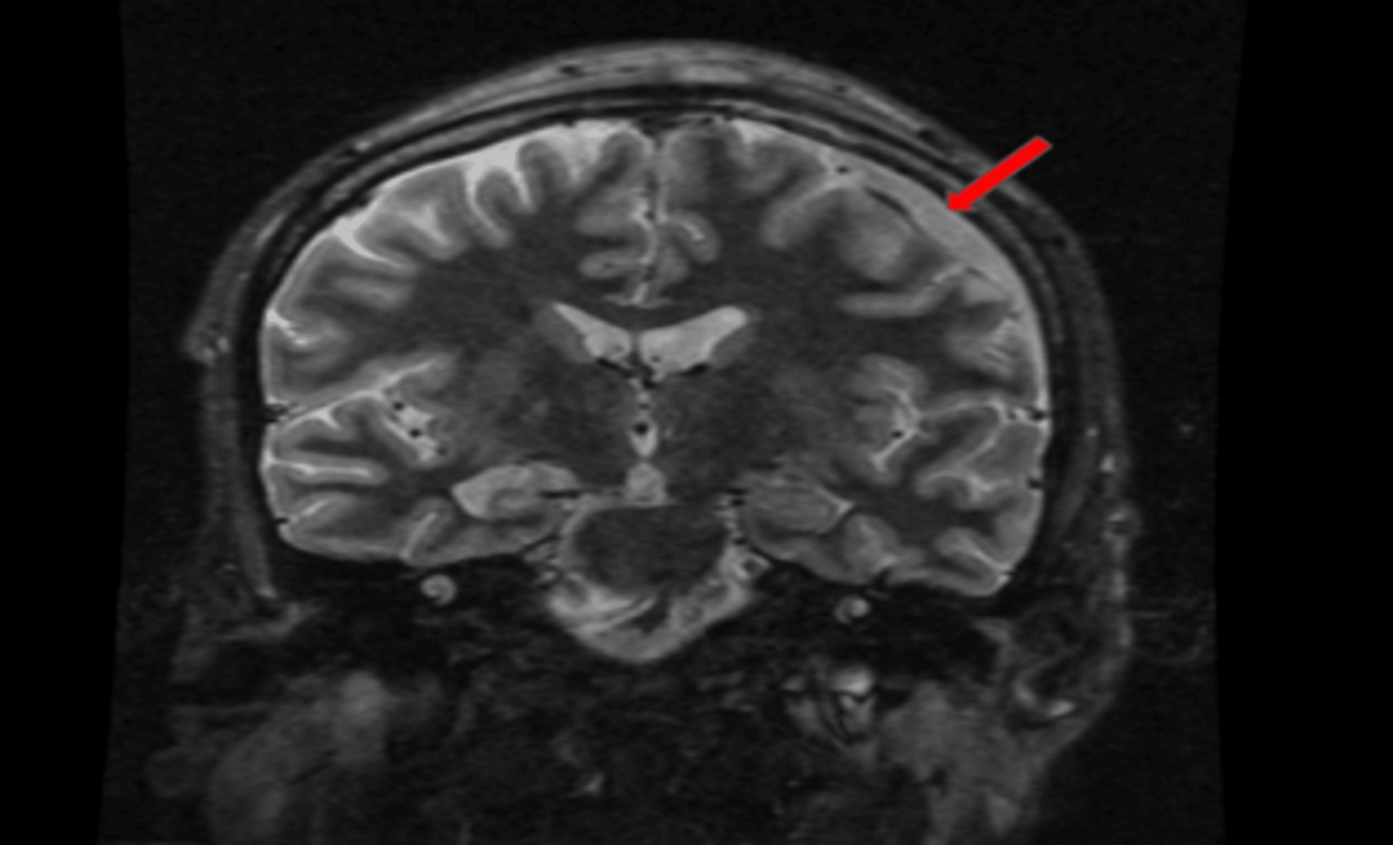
MRI of the brain. MRI of the brain showing an enhancing lesion in the subdural space (red arrows). Differential diagnoses are intracranial bleeding versus subdural empyema.
